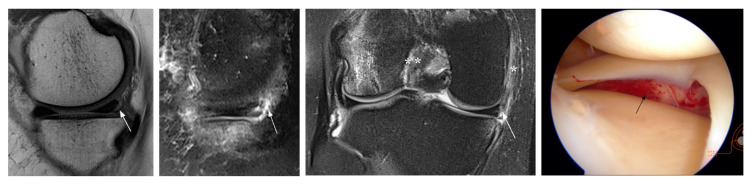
Unavoidable false-positive MRI diagnosis (patient 8). MRI shows unequivocal signs of a tear at the posteromedial meniscocapsular junction (arrows). Also note tearing at the medial collateral ligament (MCL, *) and acute ACL injury (). Arthroscopy indicates a normal medial meniscus and hematoma at the MCL (arrow).
